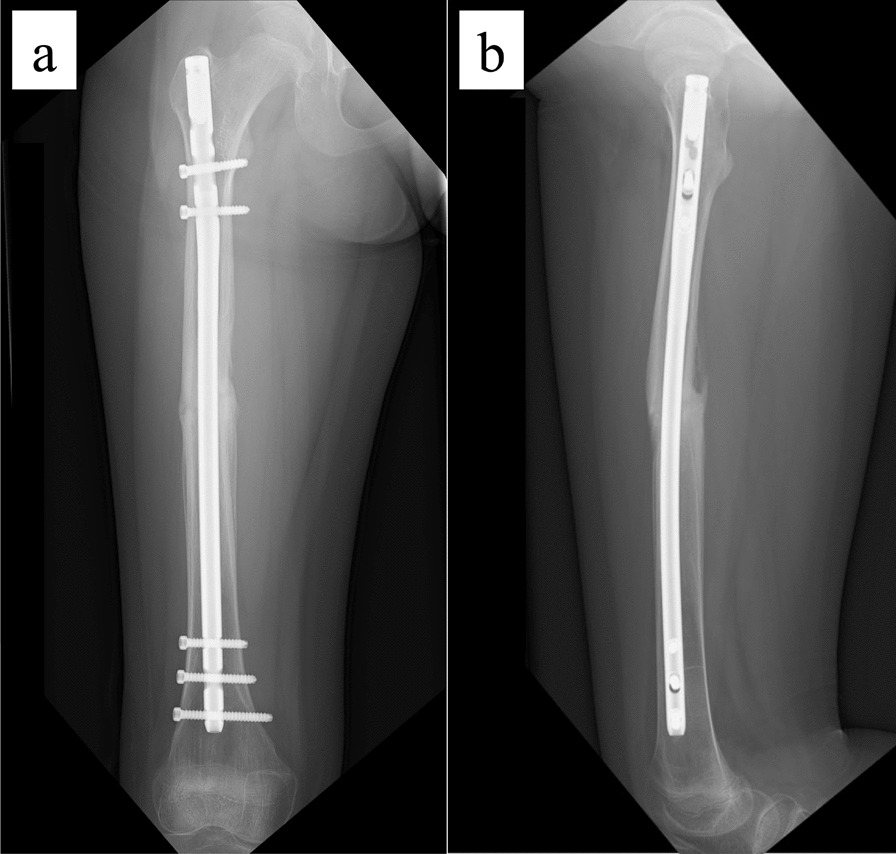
X-ray images and external views of the patient’s lower extremity 3 months after revision surgery. a Anteroposterior image and b lateral image. Bone fusion is observed in all images
